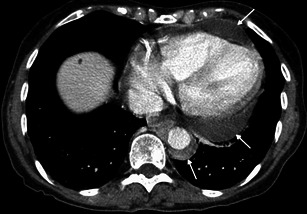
Axial enhanced CT images of the thorax in a 71-year-old female showing pericardial fluid (arrow) and periaortic infiltration (arrows)
